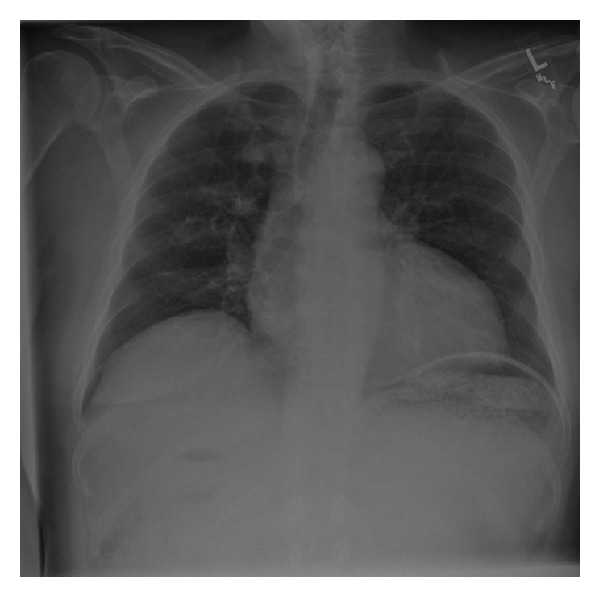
Chest X-ray of patient demonstrating enlarged gastric silhouette secondary to high-grade gastric outlet obstruction that could be mistaken for free air under the diaphragm.
